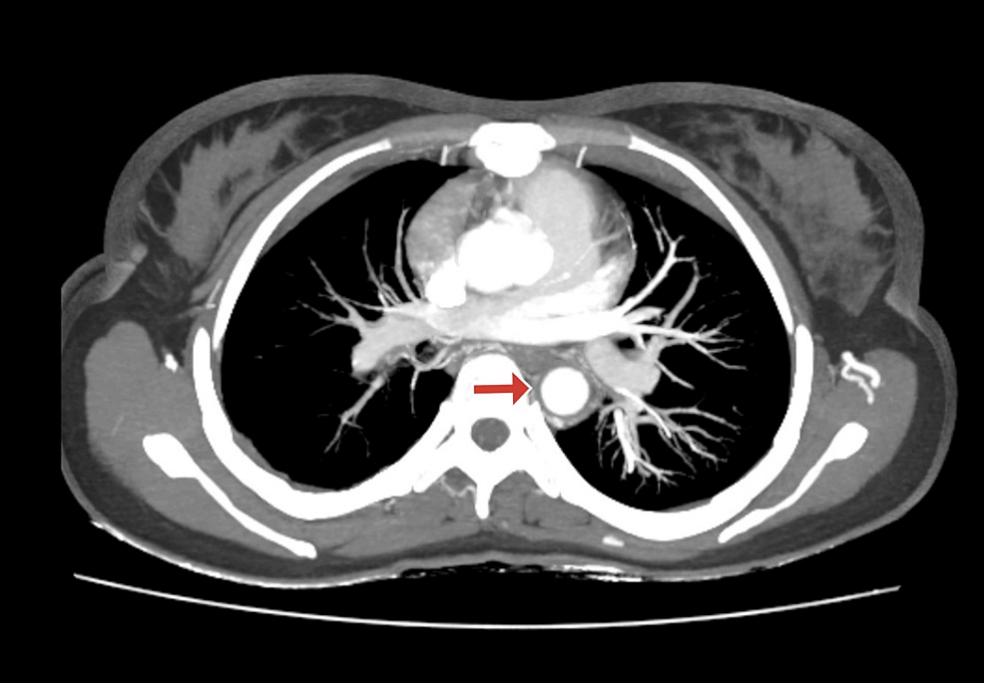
CT aortogram with the red arrow indicating the circumferential thickening of the wall of the descending aorta
